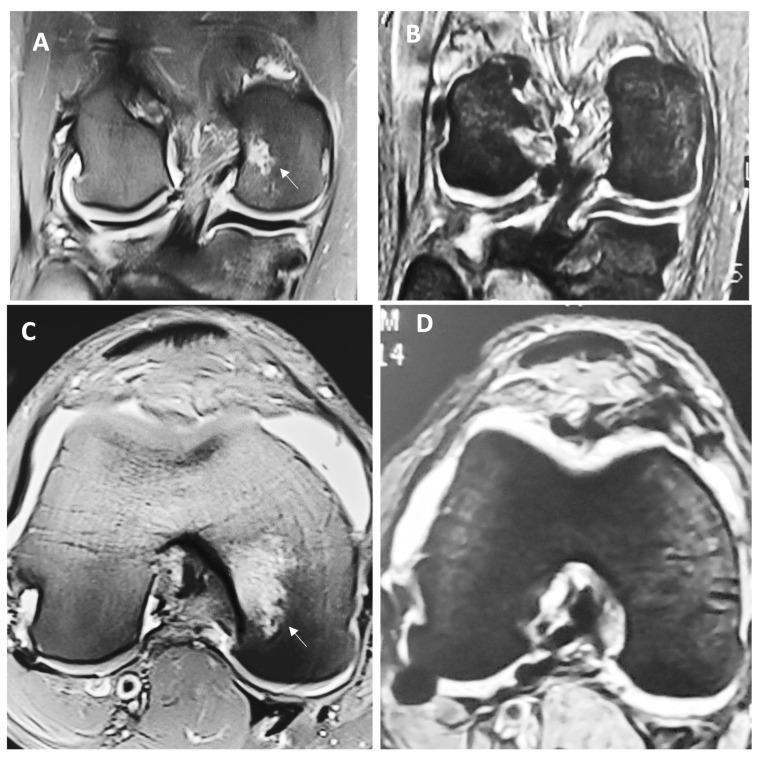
Representative MRI view of the full-thickness cartilage defect and adjacent tissue before (A) and (C) and 6 months after the surgery (B) and (D) in a patient treated with HA 3-D scaffold. Letter (A) represents the anteroposterior view of the knee, while letter (C) demonstrates the coronal view; both are showing cartilage damage with ICRS grade IV in the medial femoral condyle (arrow), with effusion and evident subchondral edema (Henderson score of 4). Letters (B) and (D) represent the same respective views 6 months after the intervention, where the lesion has visibly disappeared and reparative tissue is layering above; additionally, effusions and edema are absent (Henderson score of 1).
